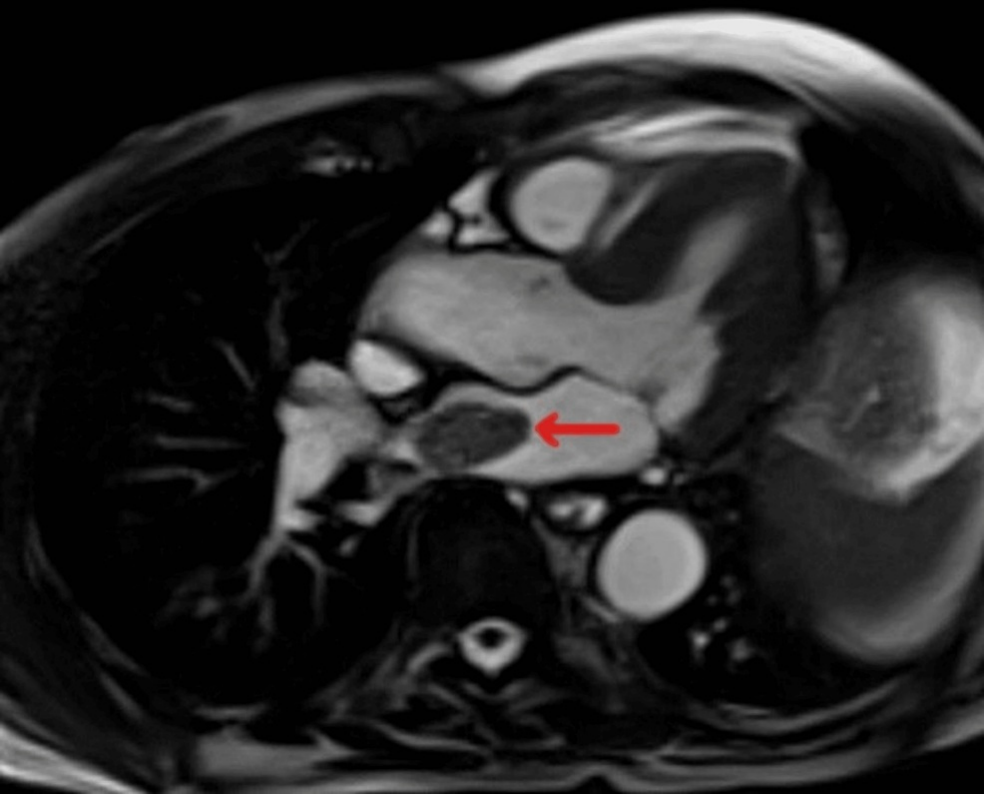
Cardiac MRI showing left atrial mass on 4C CINES image (red arrow). MRI, magnetic resonance imaging
